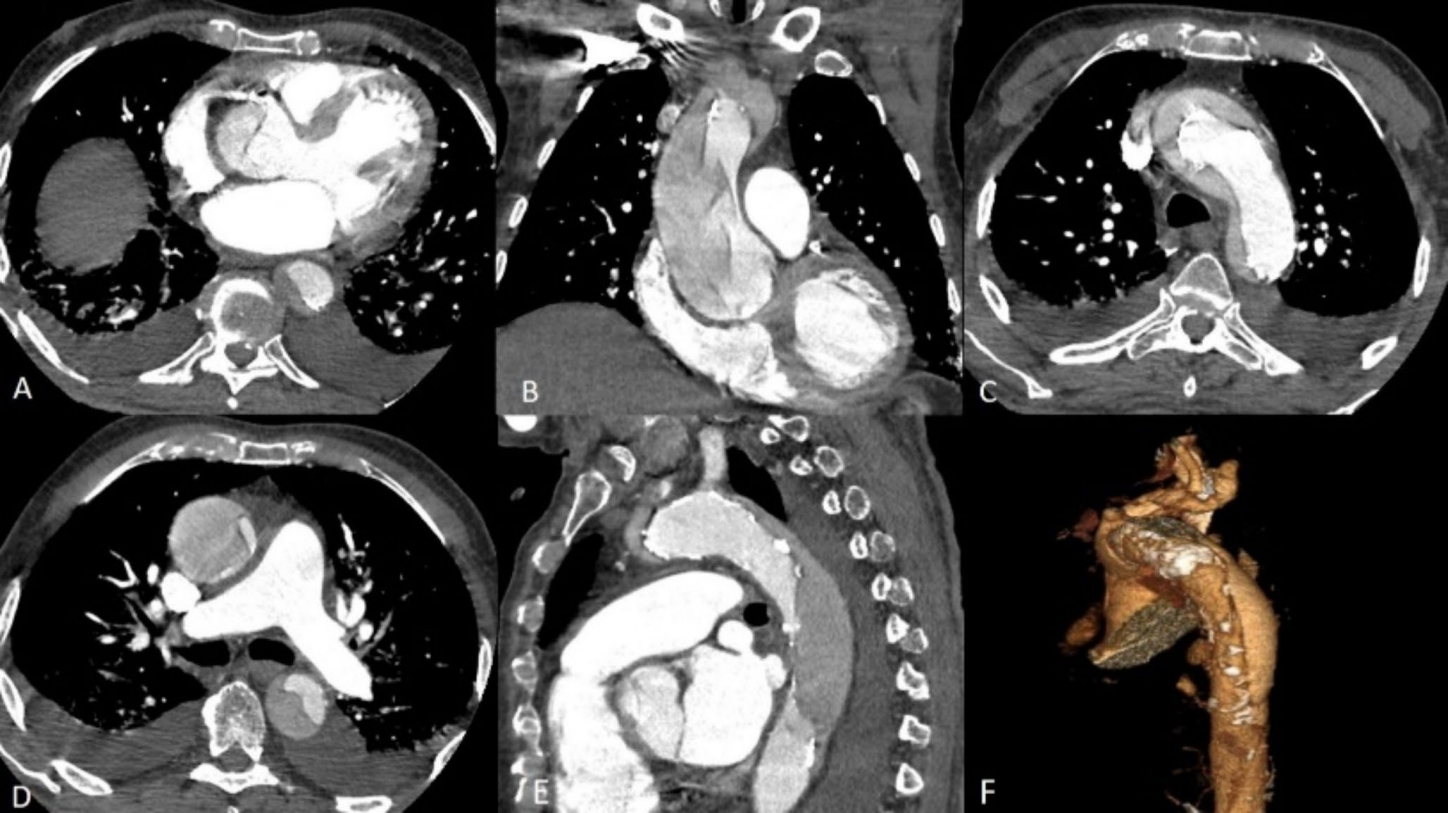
Type A aortic dissection. Axial CTA images at the level of the right coronary artery (A), at the level of the aortic arch (B) and at the level of the pulmonary artery (C) demonstrating the type A aortic dissection. Coronal (D) and sagittal (E) images reveal the extent of the dissection as it tracks longitudinally along the media layer of the aorta, thereby forming a second blood-filled channel (false lumen) within the vessel wall. Post processing with volume rendered image of the aortic dissection (F)
